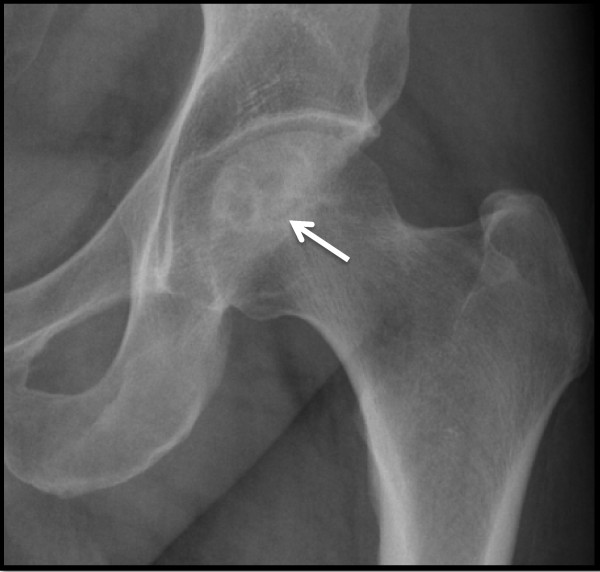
Plain radiograph in anteroposterior view (white arrow) at review four months post procedure.
